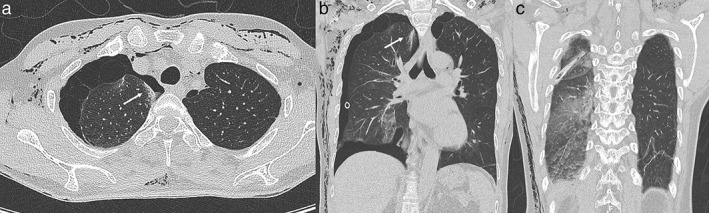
(a) Chest CT scan (coronal view) showed apical bullae and patchy areas of GGO in the right lower lobe. Another focal area of GGO is observed in the medial aspect of the right upper lobe (white arrow), (b) Chest CT scan (axial view) showed a clear view of multiple bullae and a focal area of GGO in the right upper lobe (white arrow), (c) Chest CT scan (coronal view) showed re‐expansion pulmonary edema in the right posterior lung zone manifesting with diffuse GGO and interlobular septal thickening. CT, computed tomography; GGO, ground‐glass opacity.
